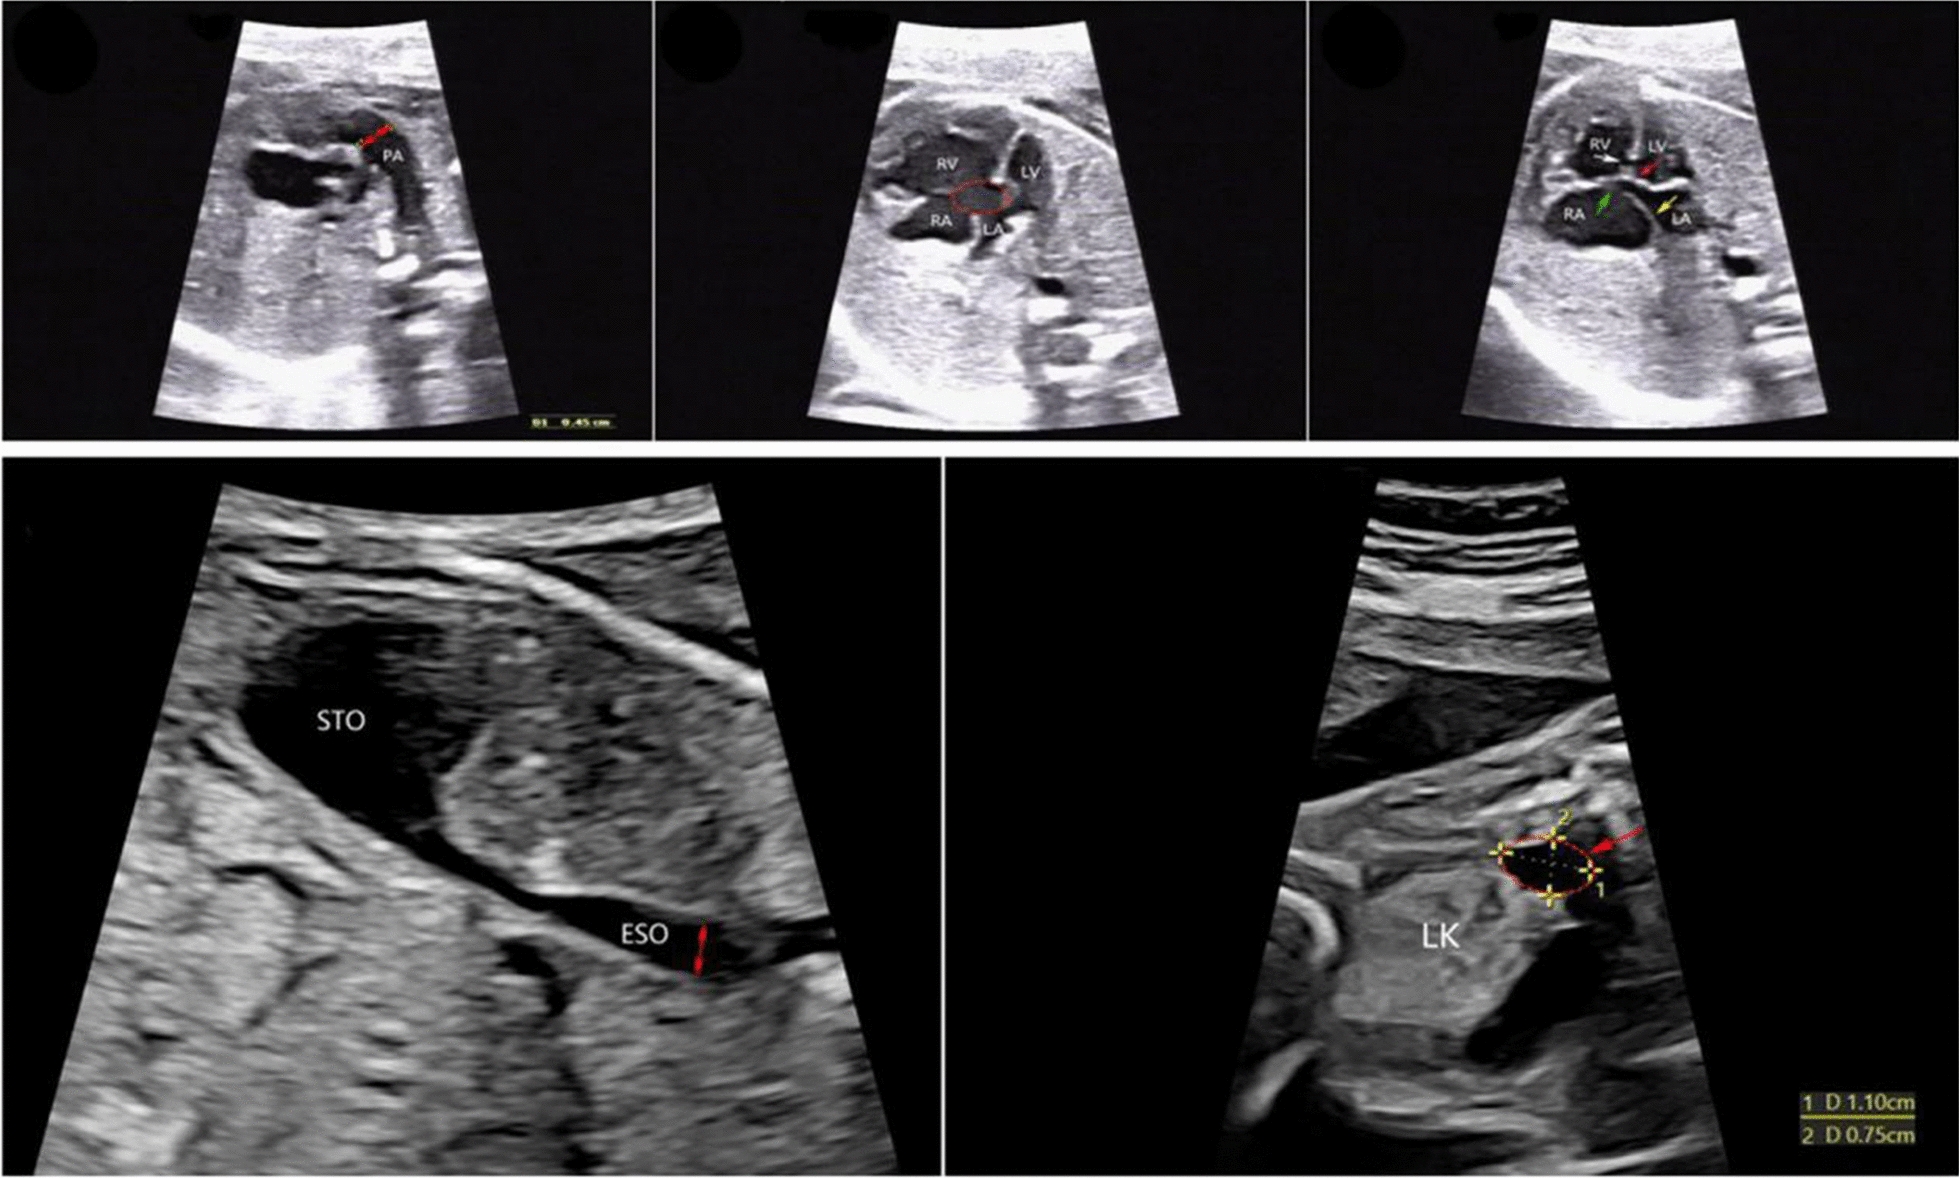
Fetal ultrasound at 23 + 5 weeks gestation showed a pulmonary artery (PA) dilatation with an internal diameter of about 4.5 mm; b complete atrioventricular septal defect manifestation during diastole; c common atrioventricular valve (red arrow), foramen ovale closure (yellow arrow), atrial septal defect with the width of 2.2 mm (green arrow), ventricular septal defect with the width of 2.6 mm (white arrow) and right heart enlargement manifestations during systole; d dilatation of the stomach measuring about 32 × 13 mm and esophageal dilation with the widest internal diameter of 9 mm (considering pyloric obstruction); e a 11 × 7.5 mm hypodense mass in the upper pole of the left kidney. Abbreviation: ESO, esophagus; LA, left atrium; LK left kidney; LV, left ventricle; PA, pulmonary artery; RA, right atrium; RV, right ventricle; STO, stomach
